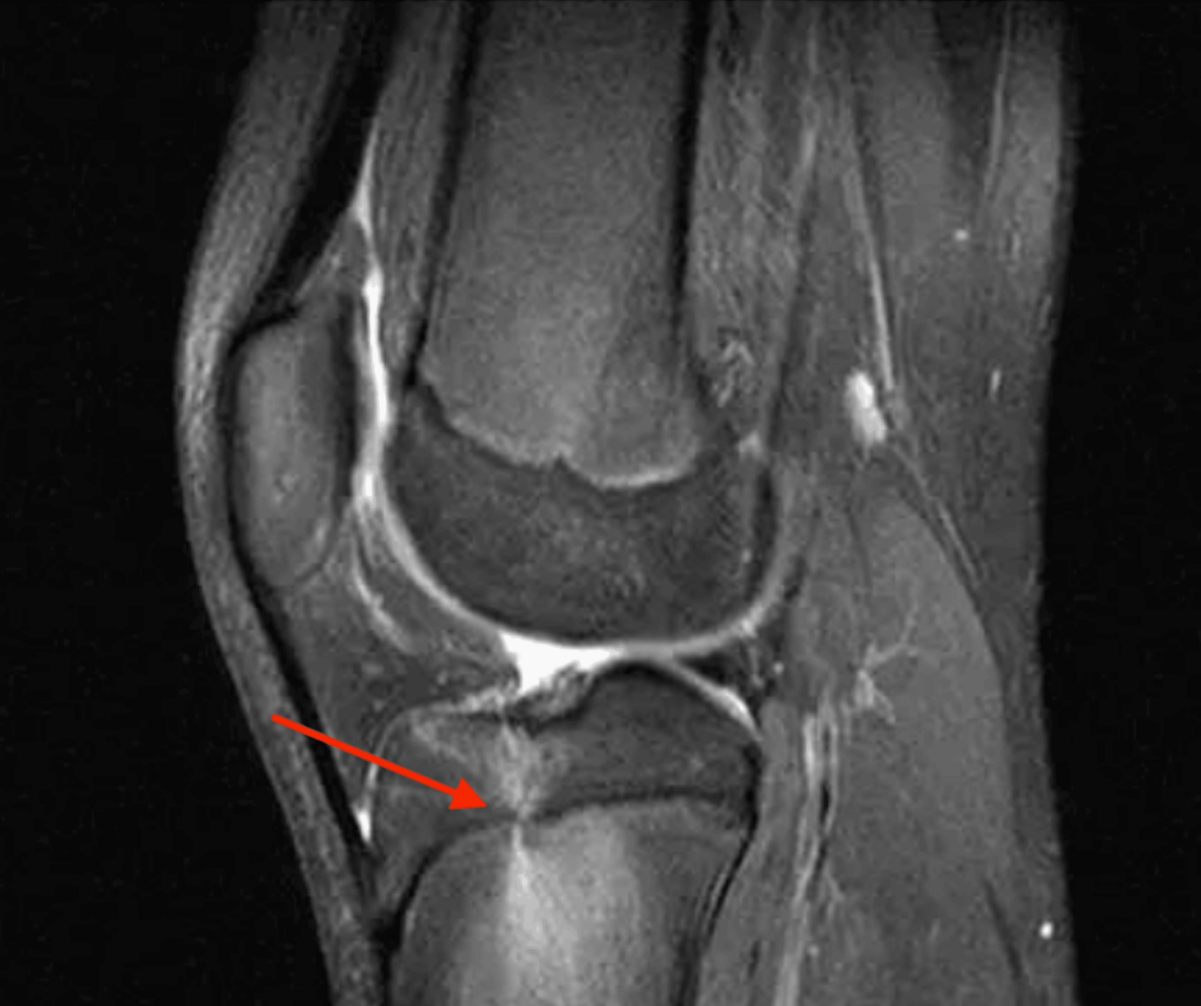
T2-weighted, fat-suppressed sagittal view of the knee showing hyperintensities in the proximal tibial physis (arrow).
